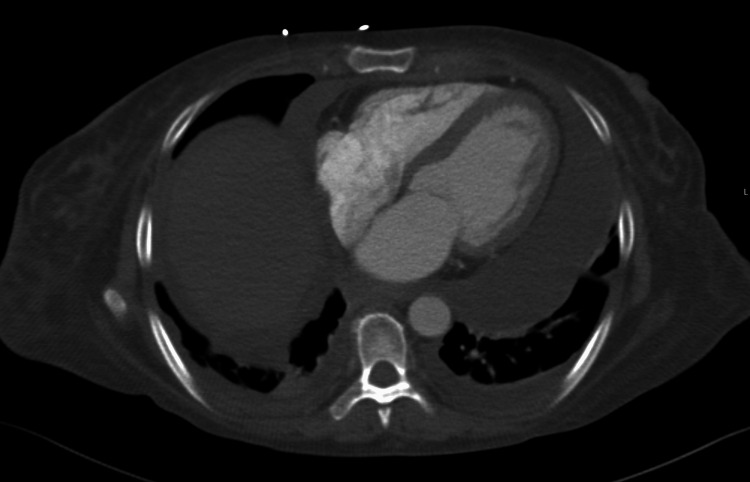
CT image of pericardial and pleural effusions
